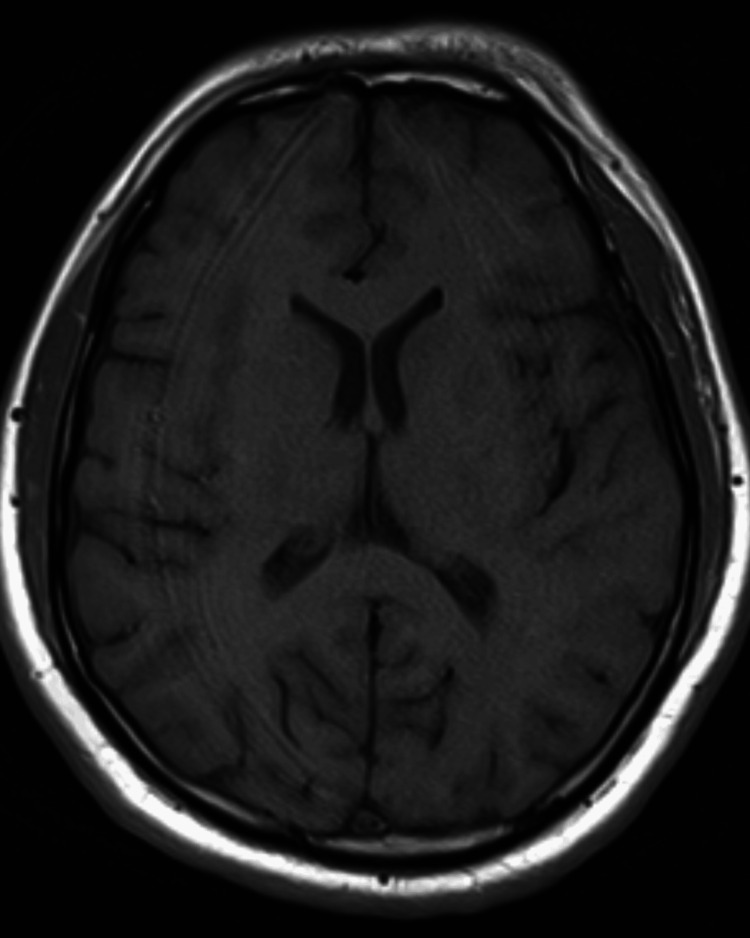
Axial T1-weighted MRI of the brain showing normal findings.
